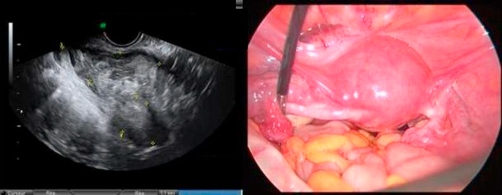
Diagnosis: (Left) 5-cm diameter cervico-isthmic gestational sac as seen on endovaginal ultrasonography; (Right) laparoscopic exploration.
